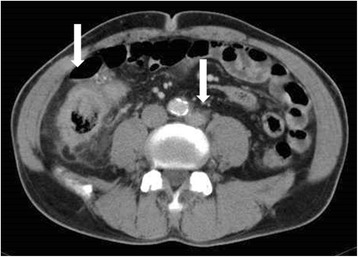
Computed tomography of case 2. An image before the operation shows para-aortic lymph metastasis and abdominal wall invasion (arrow)
